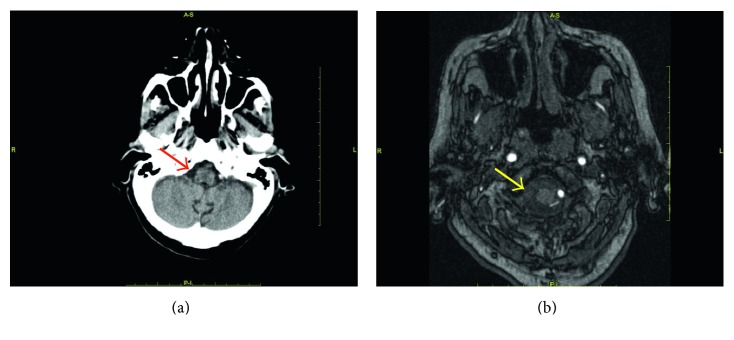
Brain CT scan (a) and MRI (b) showing the complete occlusion (arrows) of the right intracranial vertebral artery.
